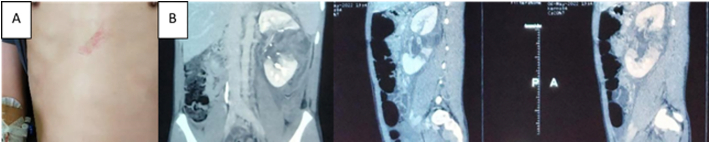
(A) Physical examination revealed a hematoma in the epigastric region; (B) Computed tomography (CT) scan revealed a total left renal rupture with avulsion of the renal hilum and devascularisation of the medial pole.
